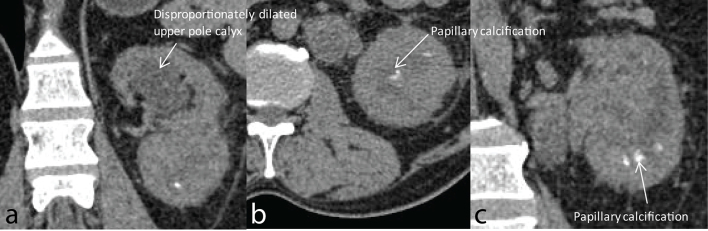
(a–c) Plain CT images axial and coronal reformats (a) showing disproportionately dilated upper pole calyces with perinephric fat stranding; (b,c) showing papillary calcifications in the lower pole.
